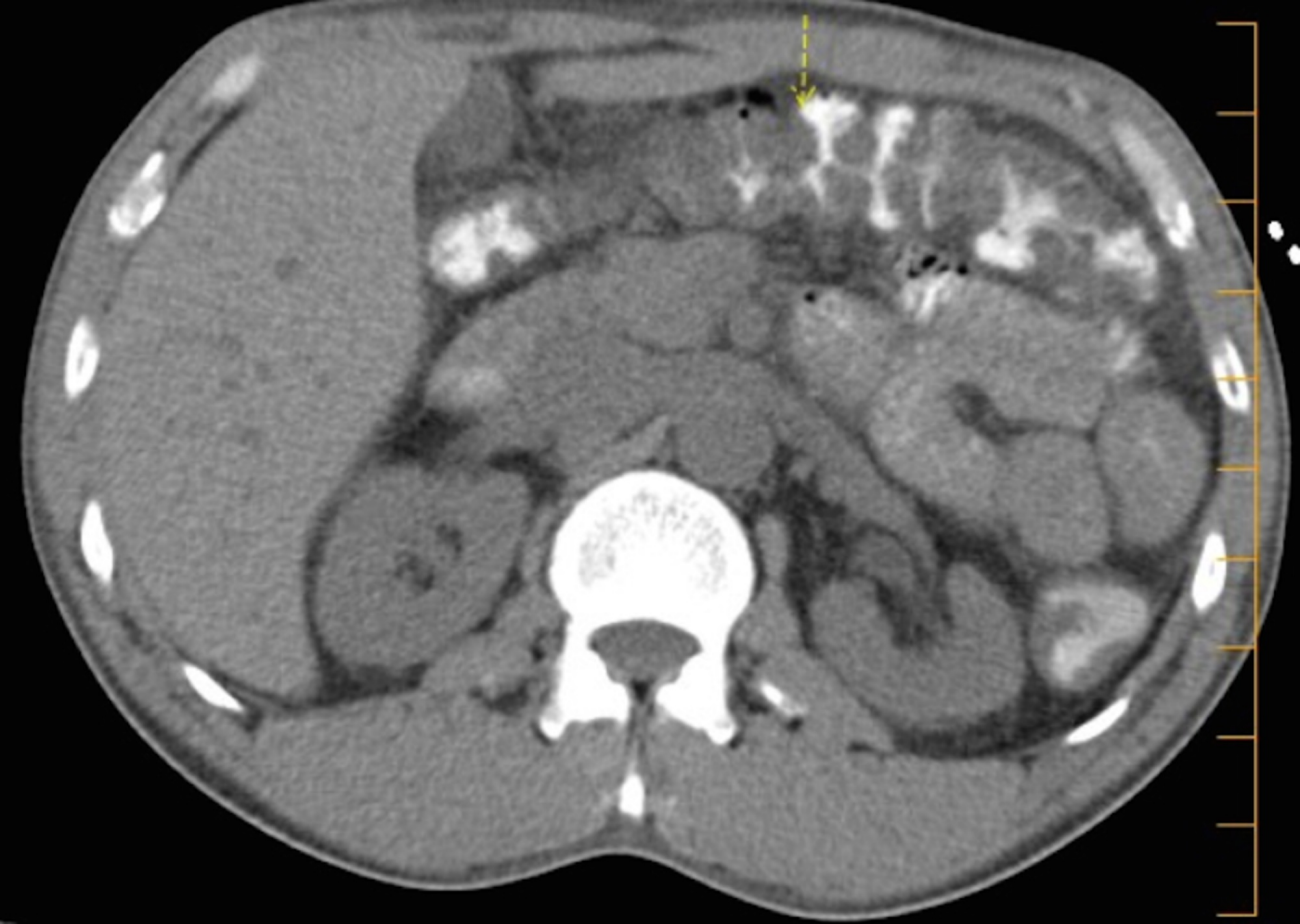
A computed tomography scan showing bowel wall thickening with “thumbprinting” and surrounding mesenteric inflammatory change
